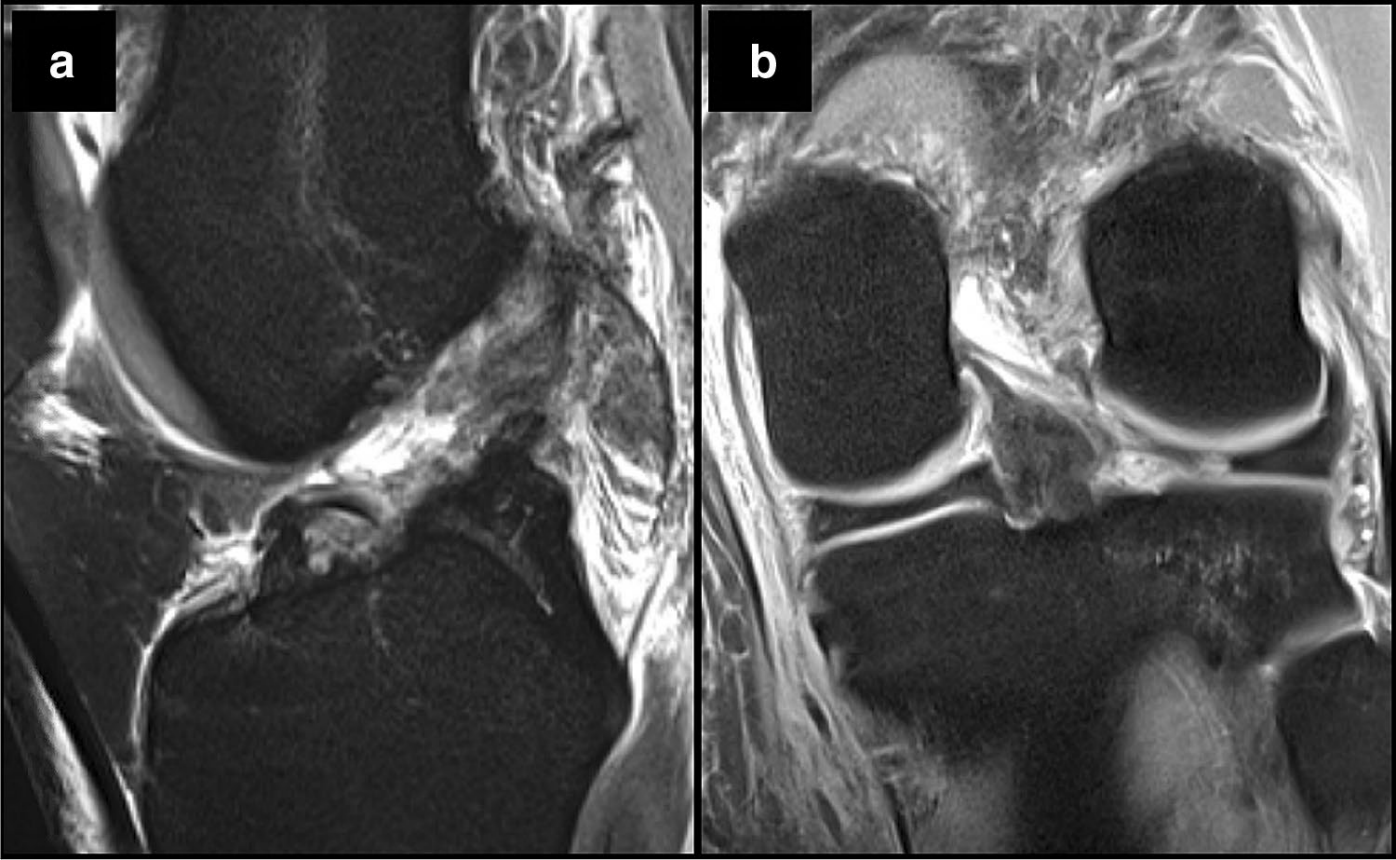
As seen in T2 MRI sequences, the patient sustained a complete ACL rupture and b associated lateral meniscus root tear
